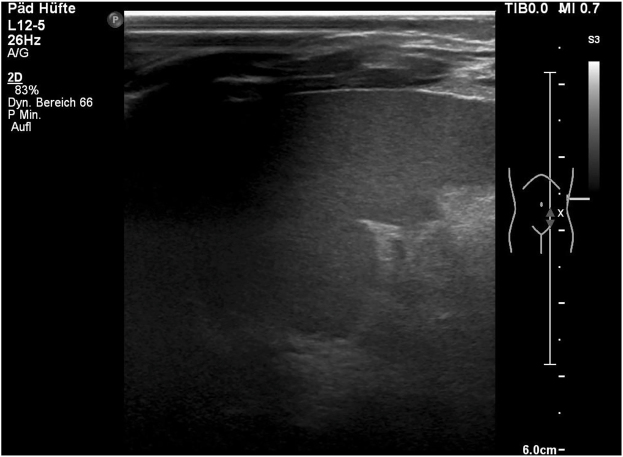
Neonatal abdominal ultrasound showing excessive abdominal fat.
